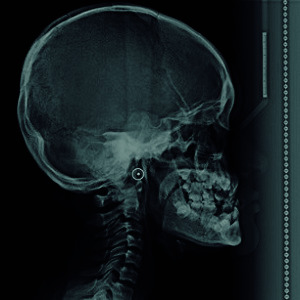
Lateral skull radiograph showing mandibular prognathism, multiple supernumerary teeth, and increased density and thickness of the contour of the cranial vault.
